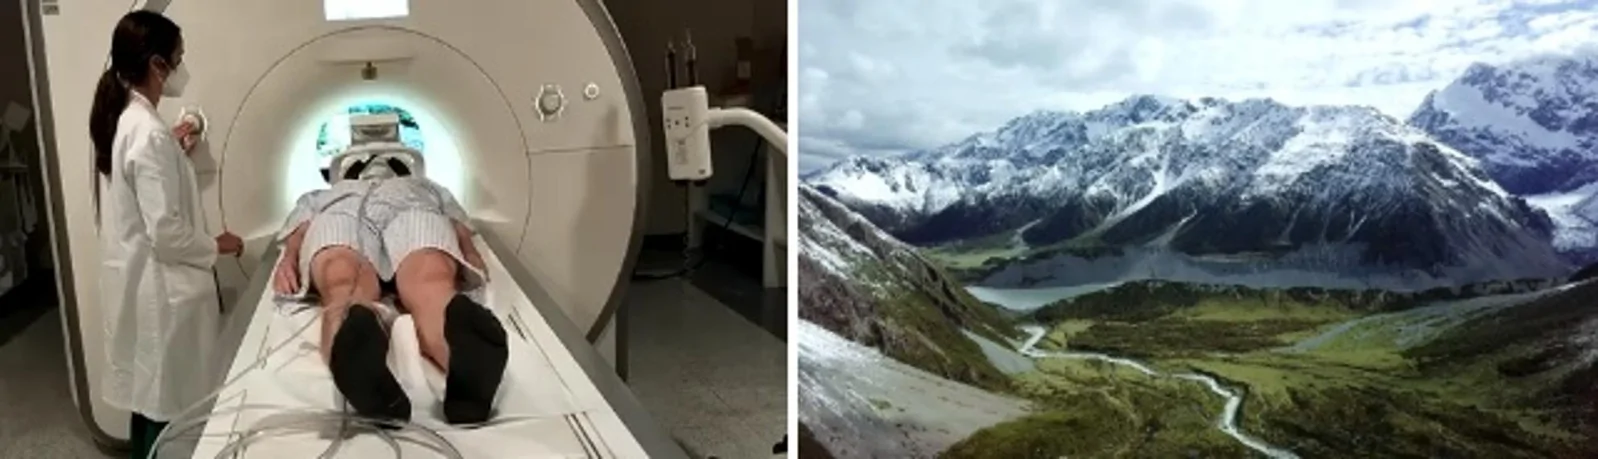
Patient preparation for WB-MRI (*left*) and an example of a natural scenario included in the video projected during the WB-MRI examination (*right*)
